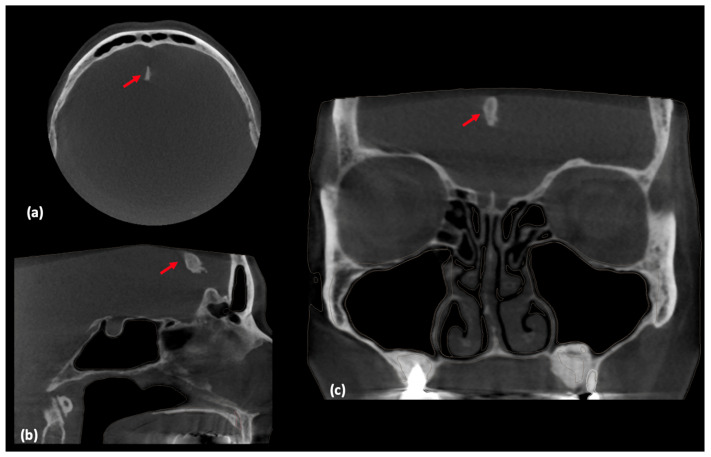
CBCT image view of the falx cerebri calcification (with the red arrow indicating the calcification). (a) Axial view. (b) Sagittal view. (c) Coronal view.
